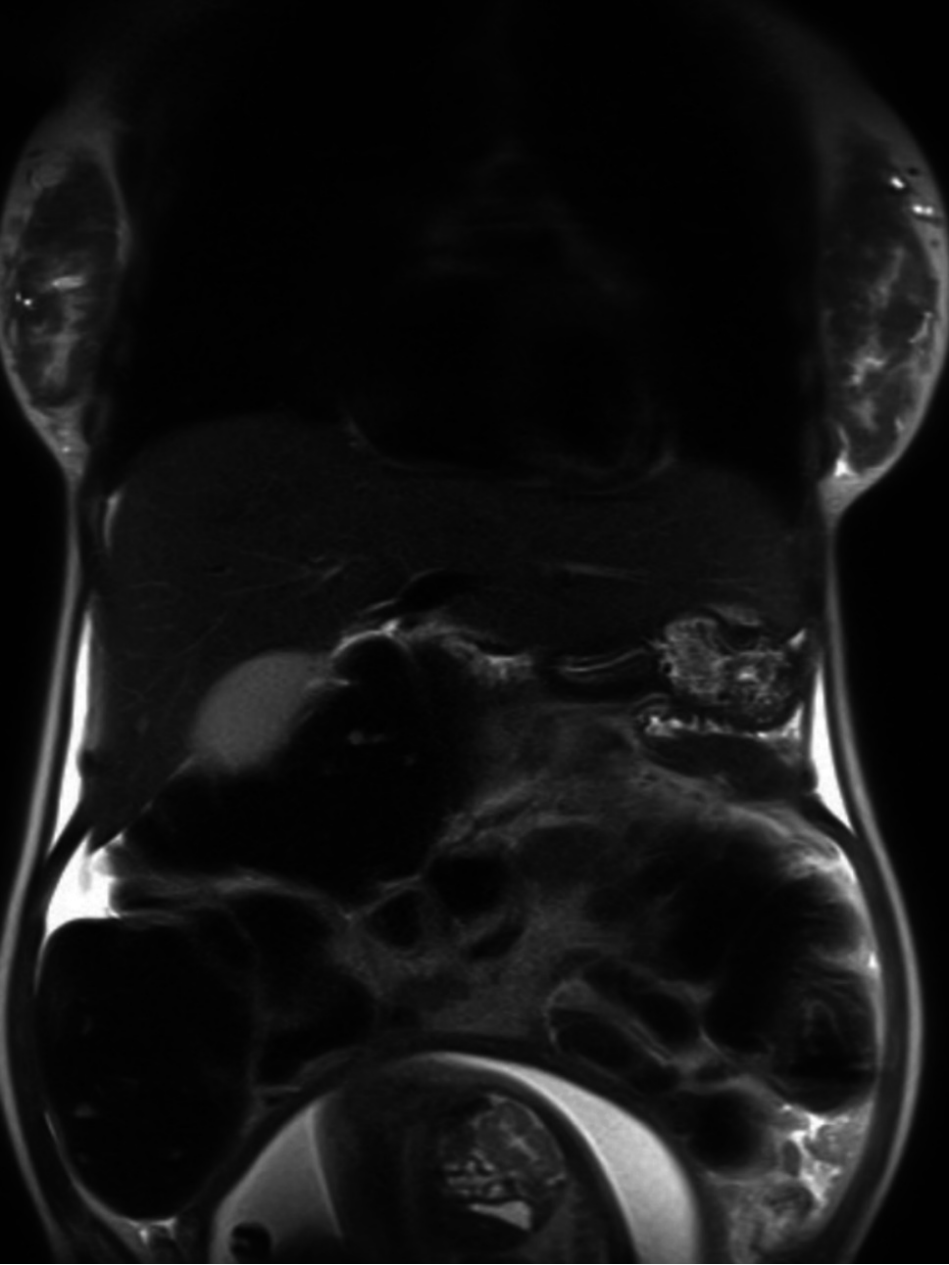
MRI of abdomen without contrast MRI showing obstruction of the distal colon with dilation of the upstream colon and retained feces. Gravid uterus is partially seen.
